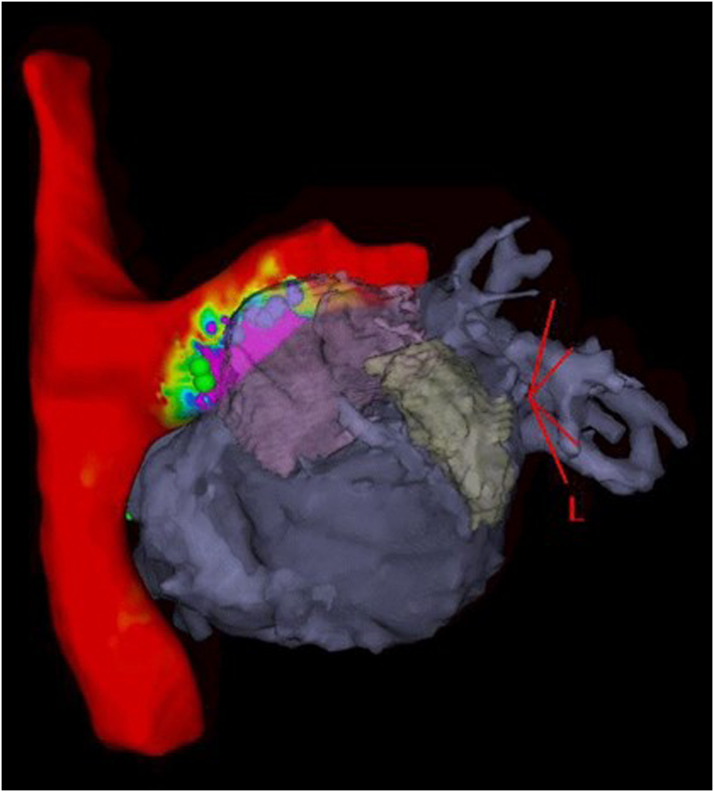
3-Dimensional Electroanatomical Map of the Left Pulmonary Artery Combined With Computed Tomography Angiogram of the Left Atrium and Right Atrium Electroanatomic voltage map of the superior vena cava, Fontan baffle, and left pulmonary artery with three-dimensional reconstruction of the common atrium with left atrial appendage and pulmonary veins showing the proximity of the atrium underneath the left pulmonary artery. Voltage map with color gradient: red = voltage <0.1 mV; purple = voltage >0.5 mV.
